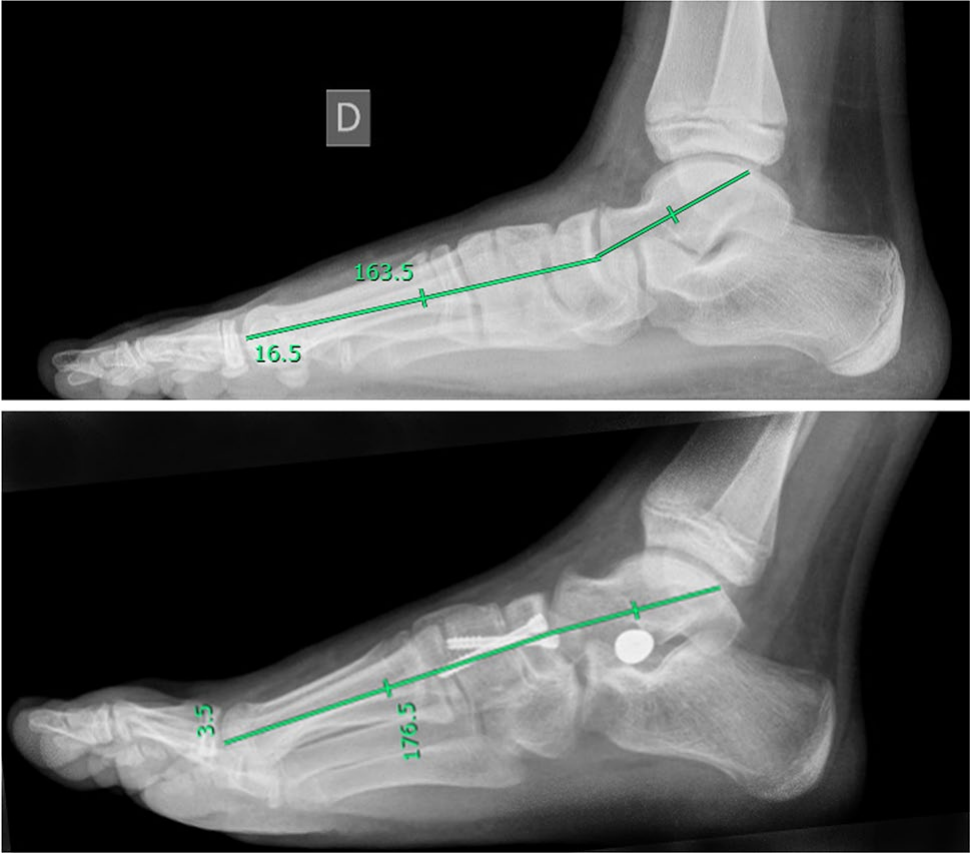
Evaluation of Meary angle in a pre (upper panel) and post (lower panel) surgical x-ray in a right foot
